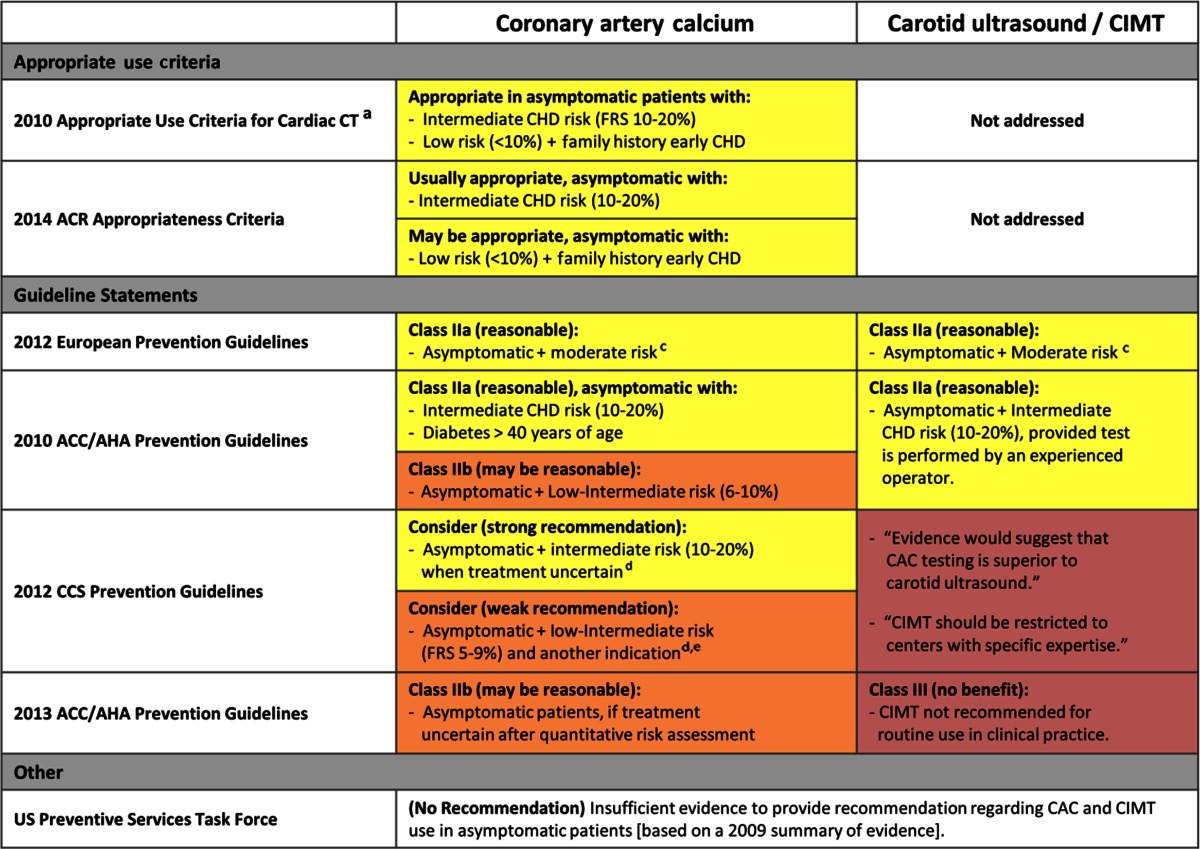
Recommendations for CAC testing and carotid ultrasound
